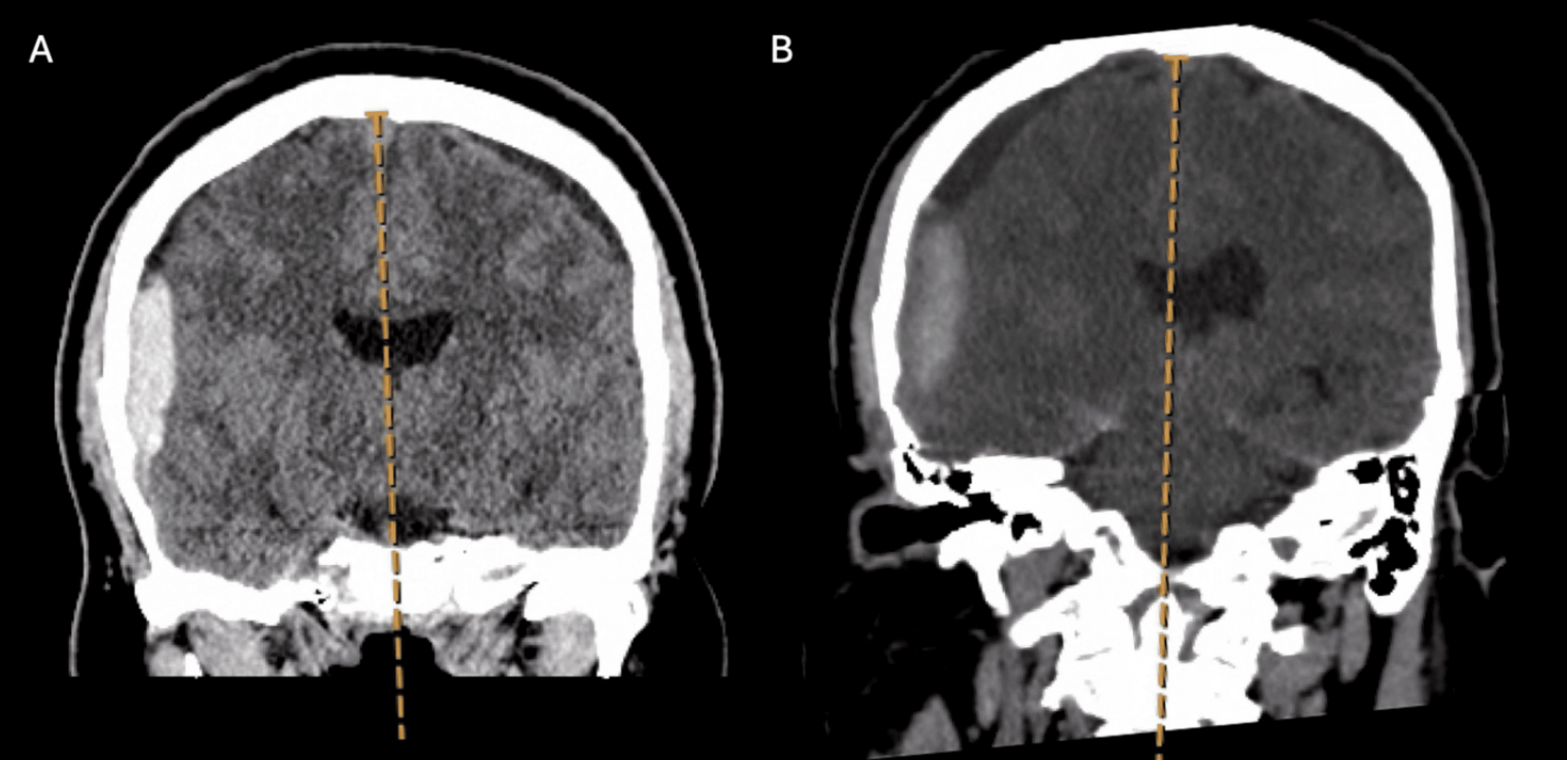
Initial and Early Repeat CT Head A: CT head on initial presentation demonstrating acute right SDH with hygroma present on left side B: Repeat CT head after decline in neurological exam demonstrating increased size of the SDH with mass effect and midline shift CT - Computerized Tomography SDH - Subdural Hemorrhage
